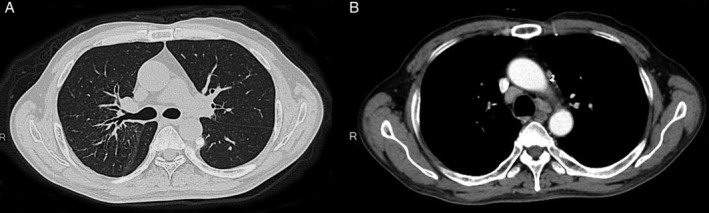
After treatment, (A) chest computed tomography (CT) and (B) contrast‐enhanced chest CT showed significantly regressive change.
